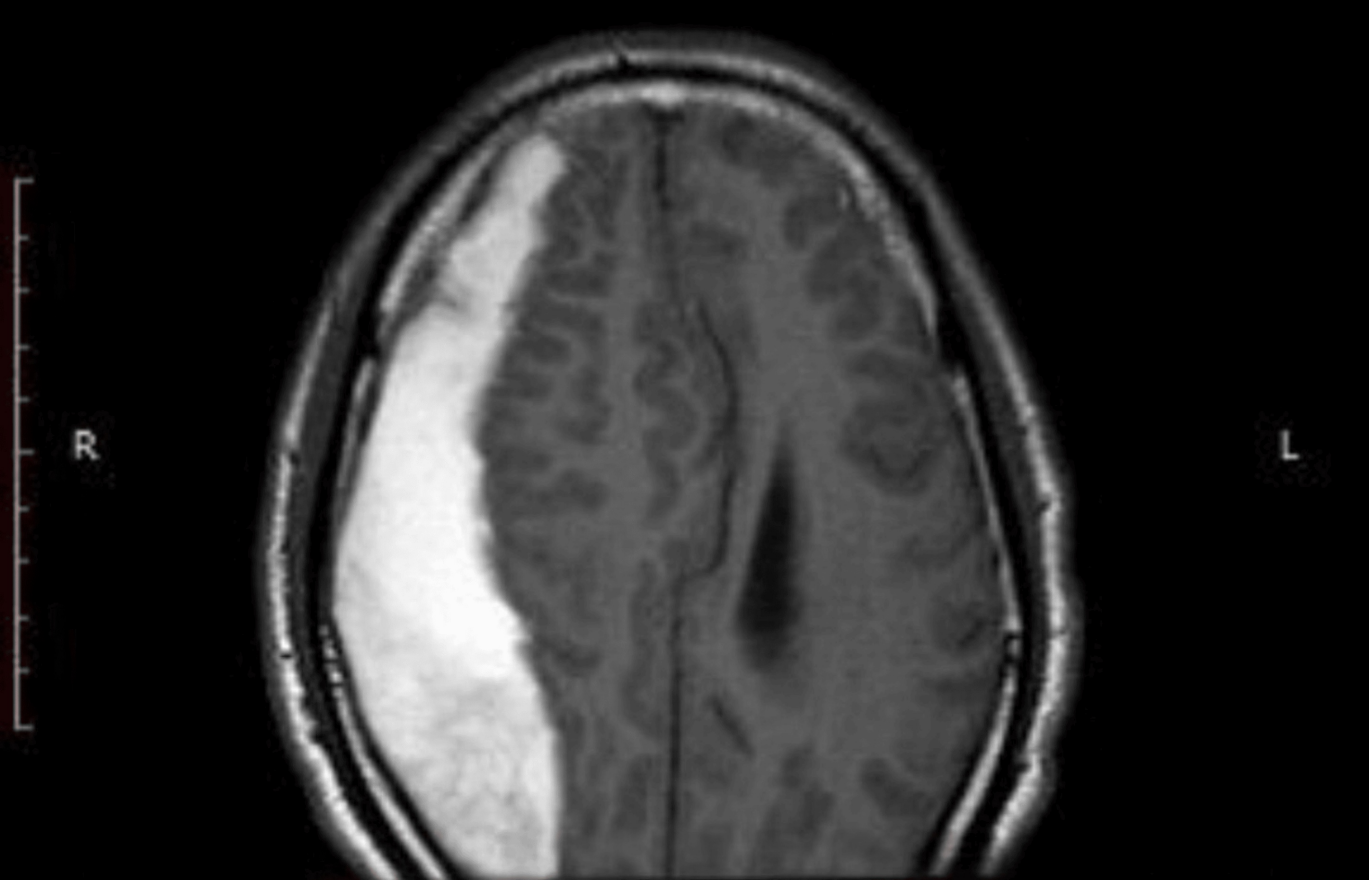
MRI showing right-sided acute subdural hematoma extending frontoparietal to occipital.
